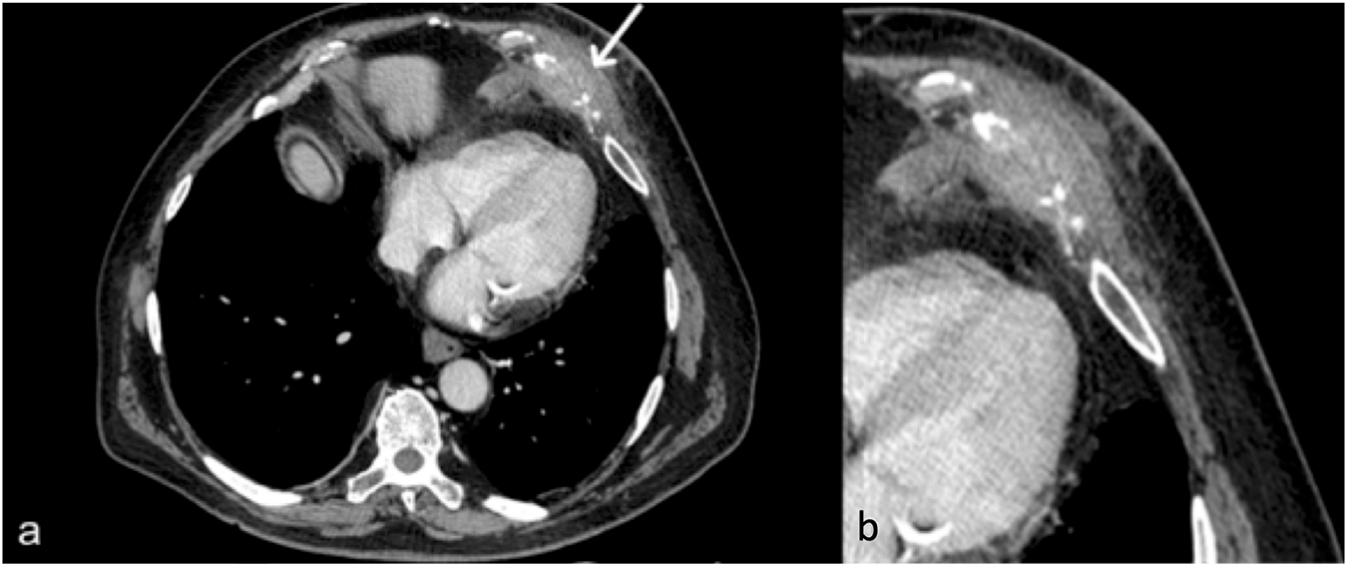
Rib chondrosarcoma in a 30-year-old male patient. a Axial contrast-enhanced CT image (venous phase) shows a soft tissue mass originating from the left costal cartilage with adjacent chondral erosion (white arrow), without extension into the underlying muscle layers. b Sagittal reconstruction confirms the cartilaginous origin and the absence of deep muscle invasion
